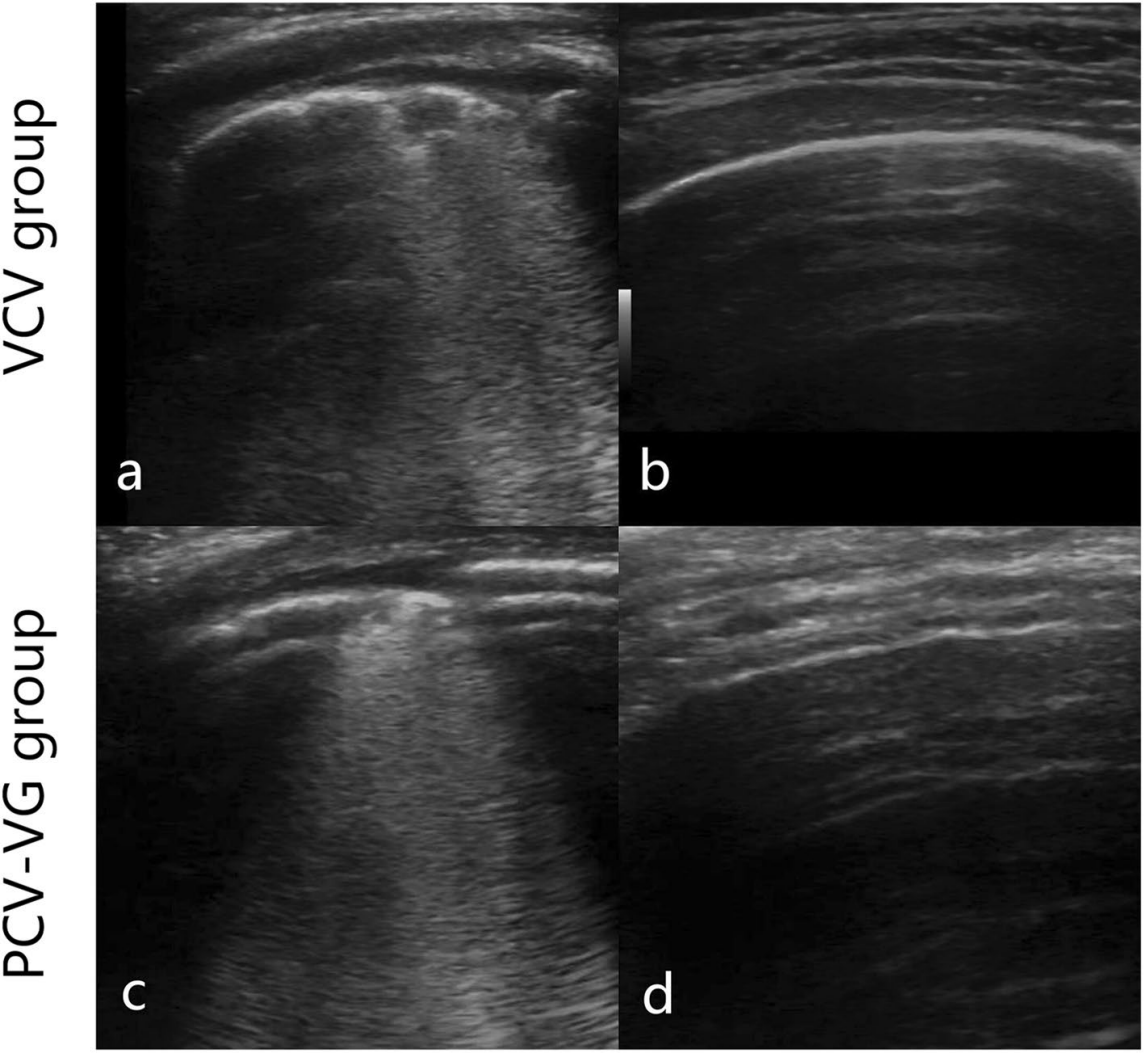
Lung ultrasound findings of dependent lung in the PCV-VG and VCV groups. The images were obtained from the posterior region of the dependent lung. (a) Lung aeration after surgery in VCV group. (c) Lung aeration after surgery in PCV-VG group. (b) Lung aeration 2.5 h after surgery in VCV group. (d) Lung aeration 2.5 h after surgery in PCV-VG group. *VCV* volume controlled ventilation, *PCV-VG* pressure controlled volume guaranteed ventilation.
